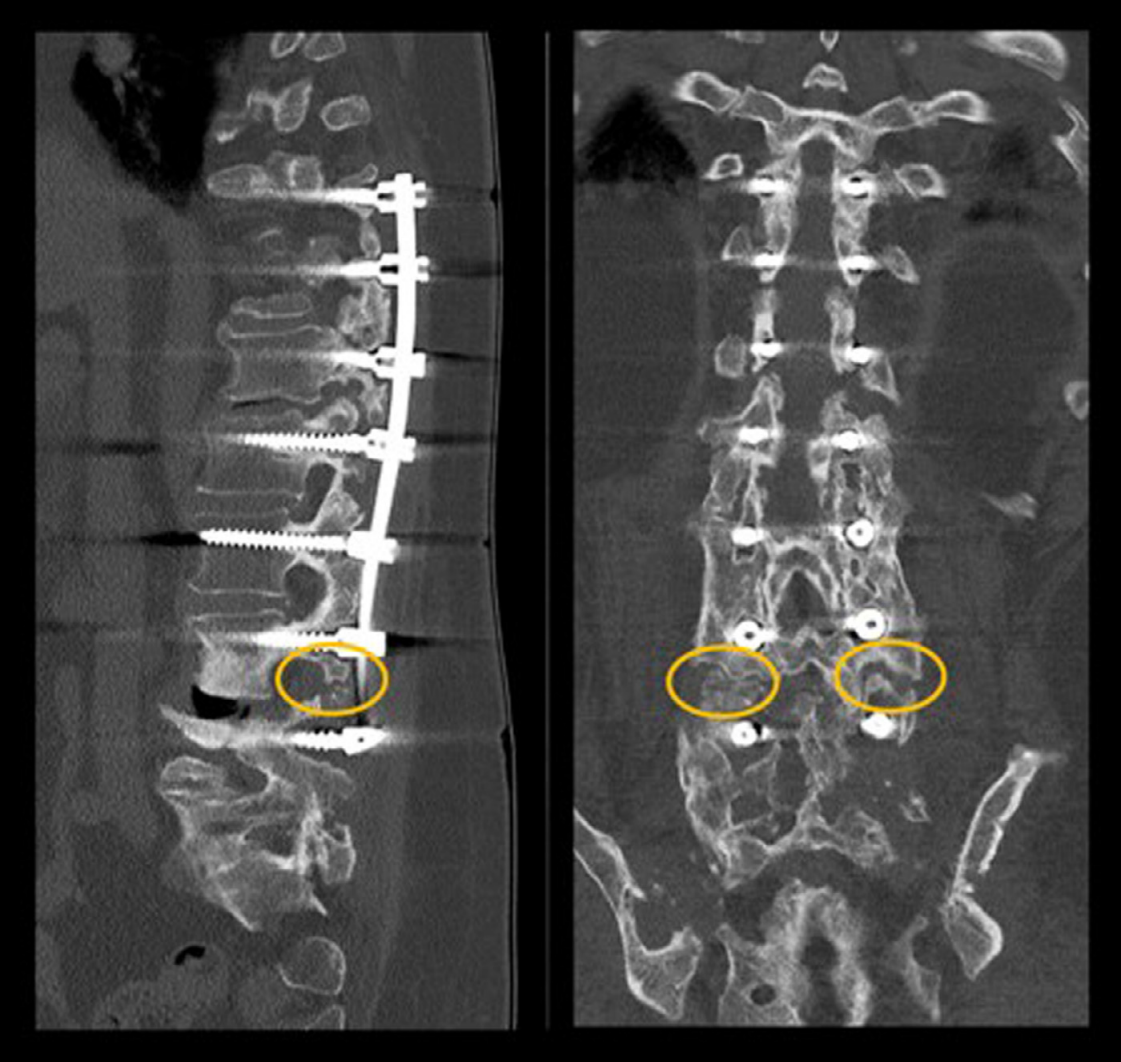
Example of a pseudarthrosis case. Lateral and coronar views of the computed tomography scan showing pseudarthroses at the level L4/5 beside large posterolateral fusion masses.
